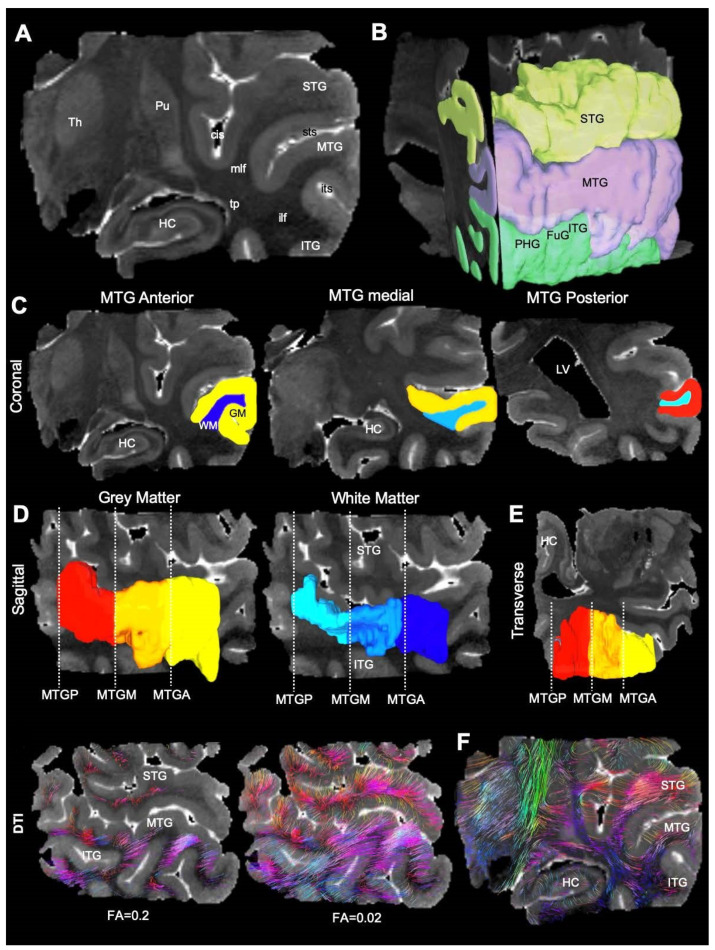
Ex vivo mesoscopic MR imaging of the postmortem non-diseased temporal lobe. (A) Coronal view through the temporal lobe reveals its internal anatomy in addition to surface landmarks, such as the superior (sts) and inferior temporal sulci (its). The circular sulcus (cis) was also evident in the coronal plane. The hippocampus, as well as the interior (ITG), medial (MTG), and superior temporal gyrus (STG) are identified in addition to white matter regions consisting of the inferior longitudinal fascicle (ilf), middle longitudinal fascicle (mlf), and the tapetum (tp). The thalamus (Th) and putamen (Pu) are also visible in this plane. (B) Segmentation of the ITG, MTG, and STG. Within the ITG, the fusiform gyrus (FuG) and the parahippocampal gyrus (PHG) are identified. (C) The MTG is divided into the anterior, medial, and posterior parts. A further distinction is made between the cortical mantle and the white matter within the MTG. (D) Three-dimensional rendering of the grey matter of the cortical mantle versus the white matter of the sagittal view plane of the MTG. (E) Transverse view of the MTG. (F) A high FA value (=0.2) typically used to trace white matter tracts visualizes streamlines confined to the white matter, whereas a lower FA termination value (=0.02) affords tracing of fiber tracts from the white and grey matter. Applying a low FA termination threshold affords the visualization of an extensive network of connections in the temporal lobe.
